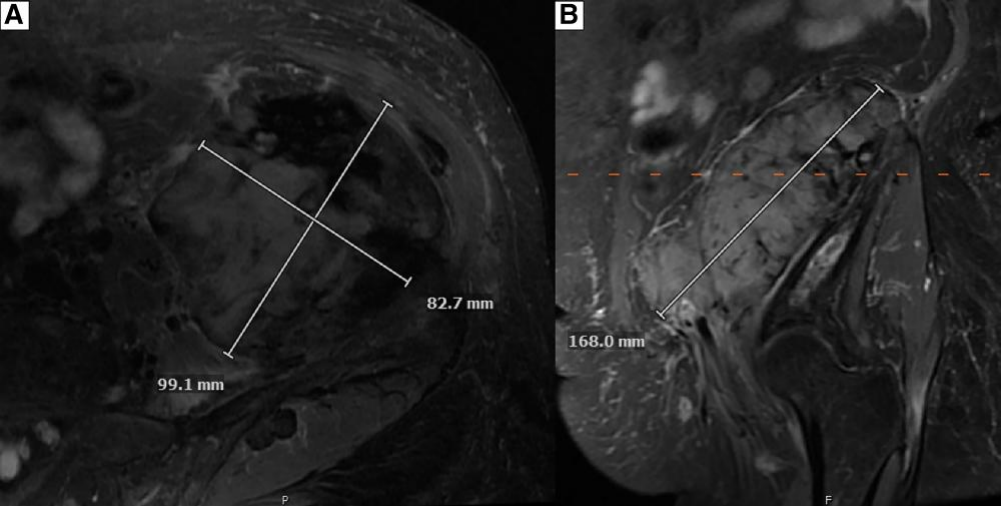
Magnetic resonance imaging (MRI) of the pelvic mass at 8 months before hospitalization with hypercalcemia. (A) transaxial (B) craniocaudal.
